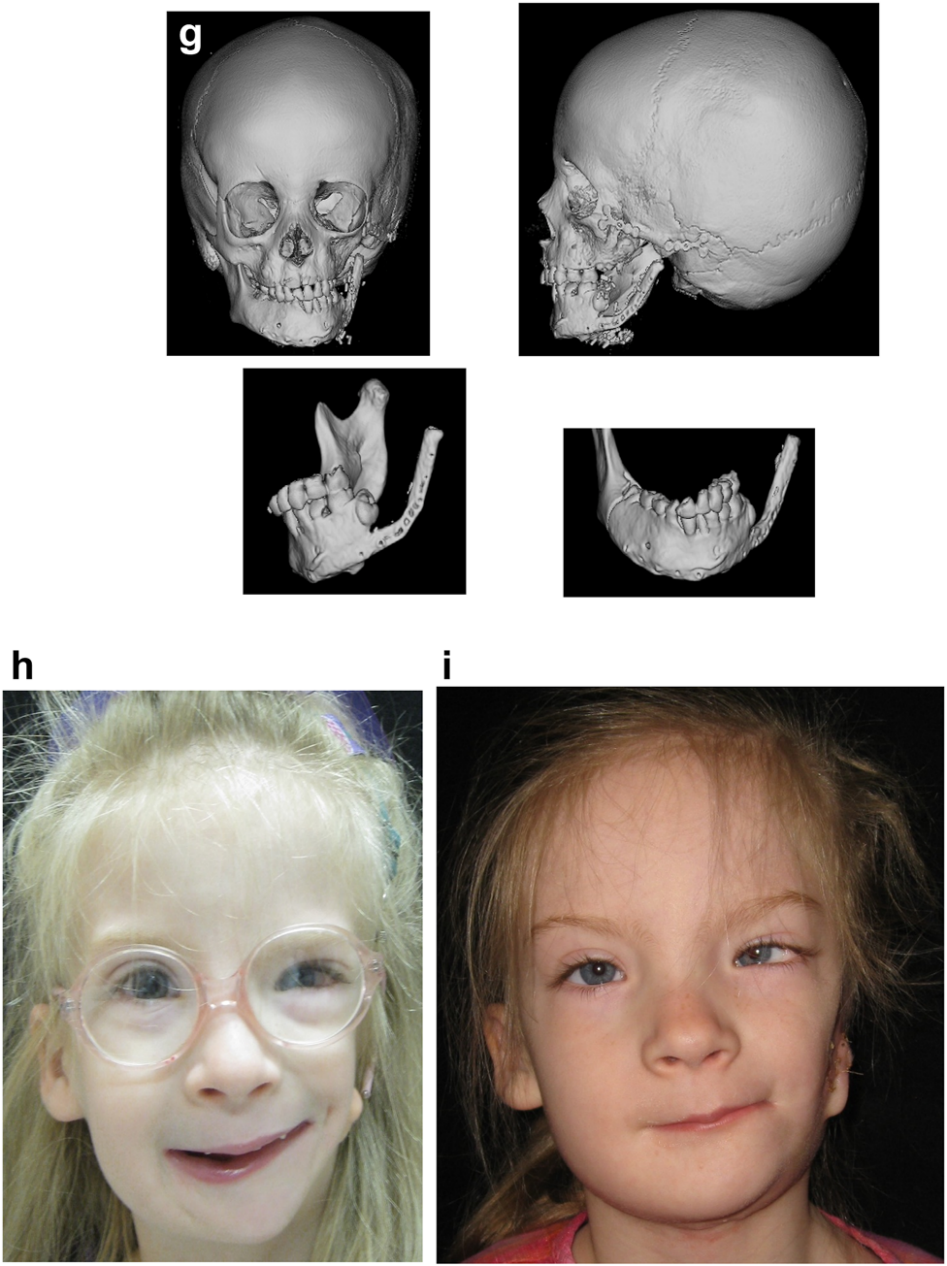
Construction of mandible with fibula free microvascular flap (g) 3D CT Outcome of the construction. (h) Pre-op. (i) Post-op.
